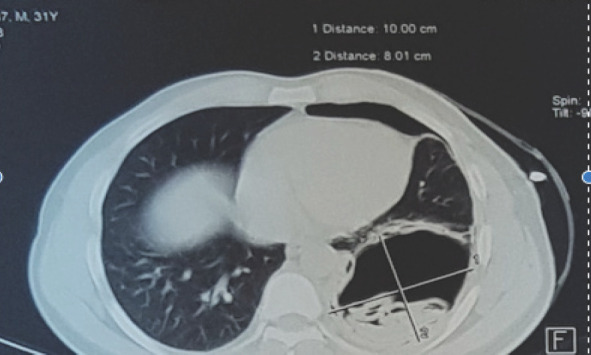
CT chest showing hydatid cyst of the lung.
